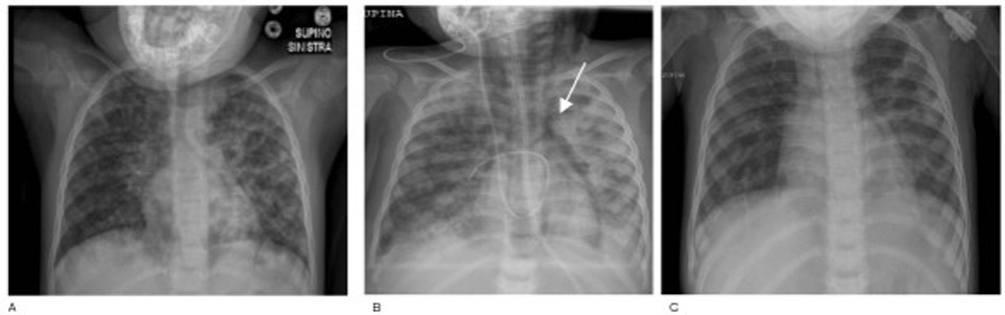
Chest X-ray film sequence. (A) Day of admission to the intensive care unit; (B) before extracorporeal membrane oxygenation with evidence of pneumomediastinum (white arrow), in spite of protective ventilatory strategy; (C) before intensive care unit discharge showing a complete resolution of acute respiratory distress syndrome.
